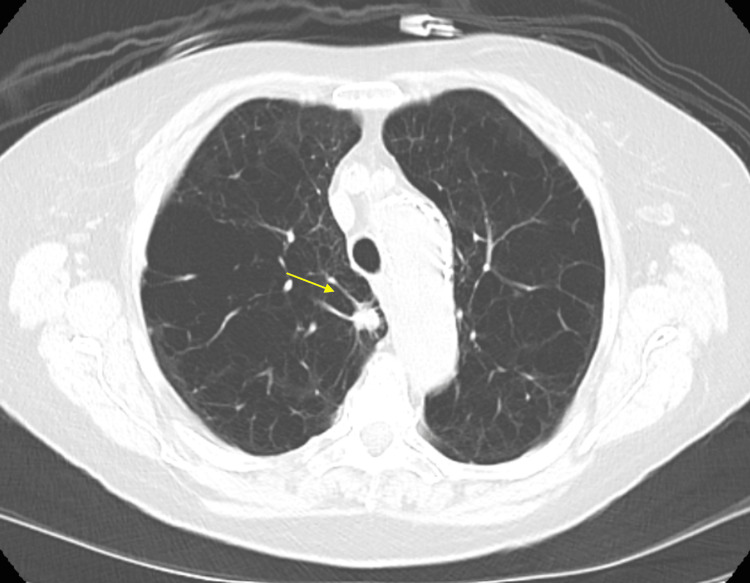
Chest CT scan in October 2024 showing a spiculated 13 x 12 mm nodule in the upper lobe of the right lung CT: computed tomography
